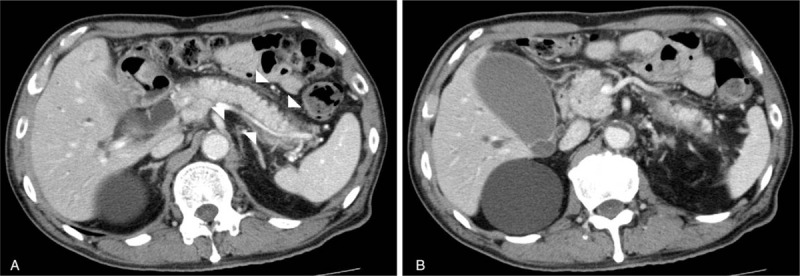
An 82-year-old man with autoimmune pancreatitis. (A) Contrast-enhanced CT showed diffuse pancreatic swelling with capsule-like rim (white arrowhead). (B) Gallbladder enlargement and intrahepatic bile duct dilation caused by constriction of the common bile duct.
